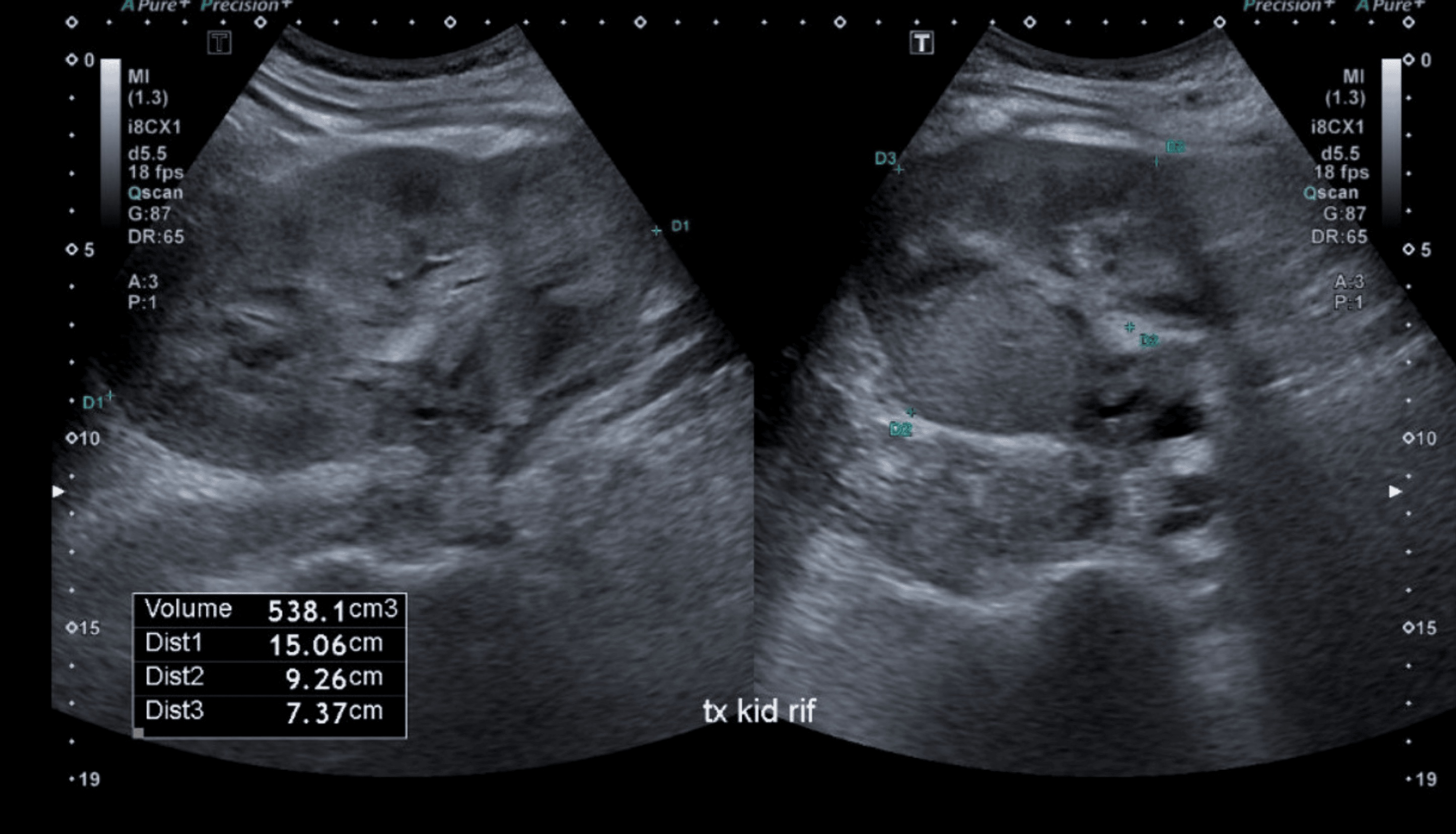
Case 2: Abdominal ultrasound The imaging shows an enlarged transplanted kidney with an approximate volume of 538 cc.
